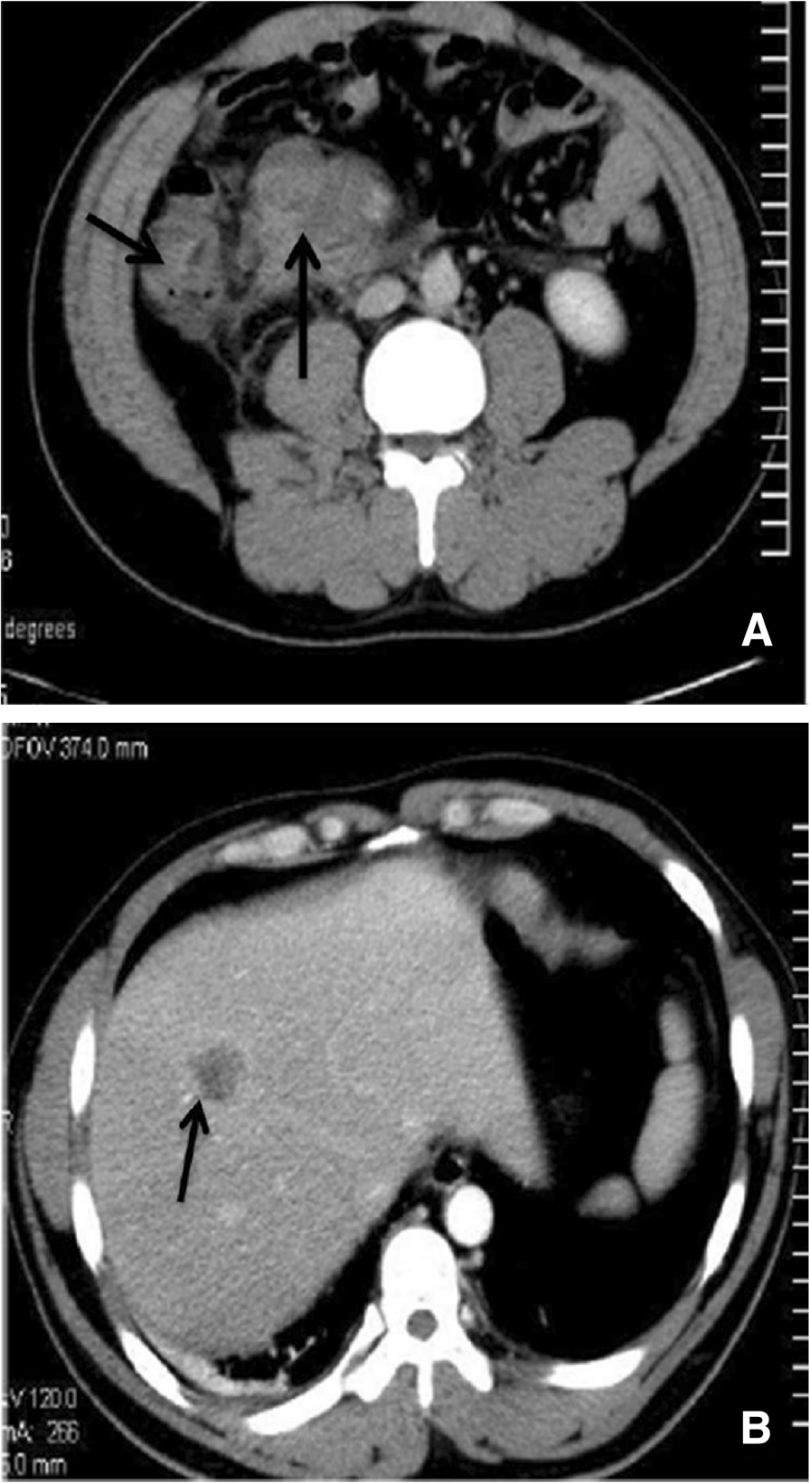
Computed tomography. (A) Note the thickened wall of the ascending colon (short arrow) and enlarged lymph nodes in the mesentery (long arrow). (B) The hepatic lesion manifested ring-shaped enhancement (arrow).
